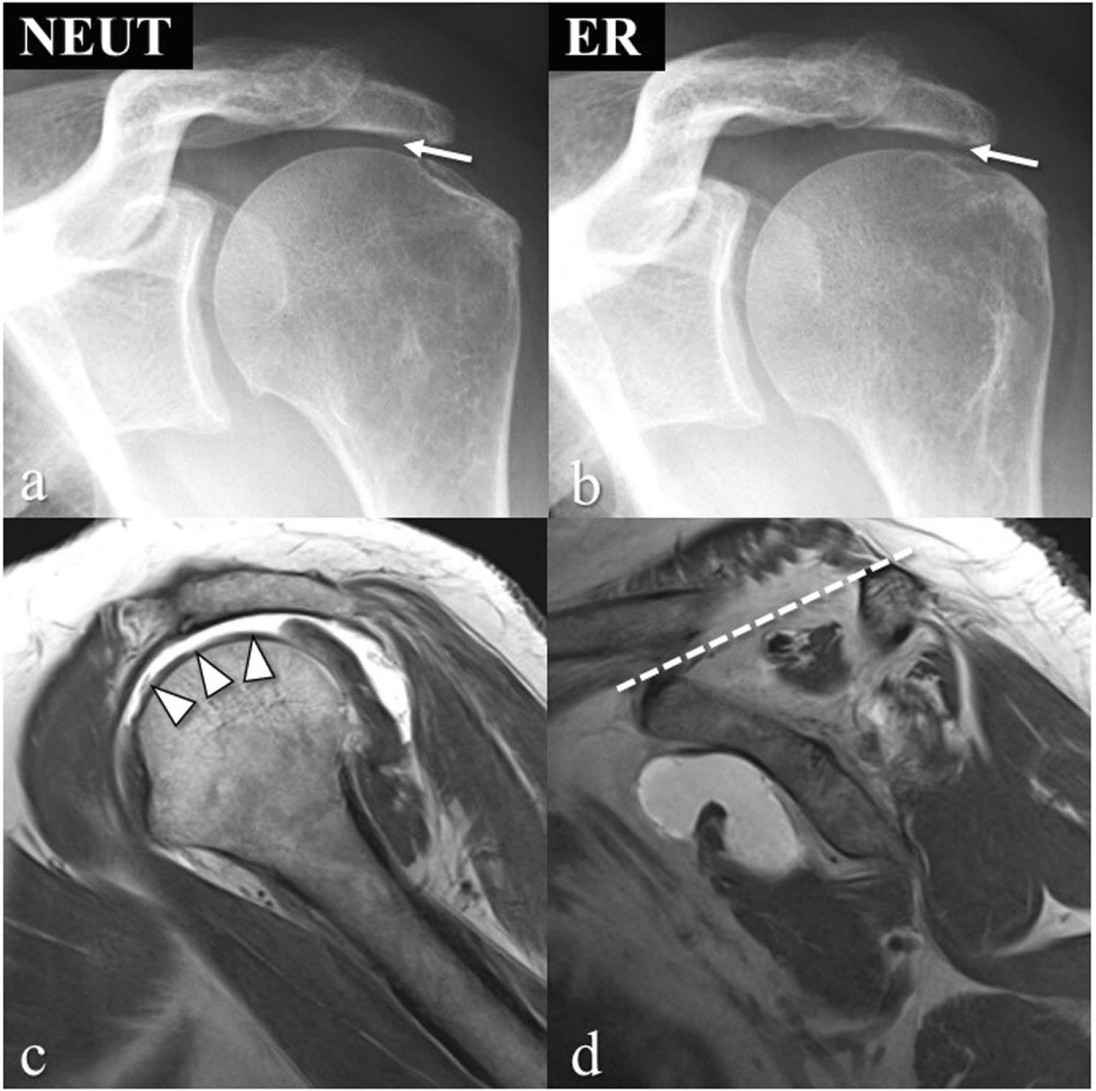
Massive rotator cuff tear in the left shoulder of a 56-year old woman. a, b Oblique anteroposterior radiograph in neutral position (NEUT) shows small acromiohumeral distance (ACHD) as indicated by the arrow, with no change in external rotation (ER). c Sagittal T1-weighted MR image depicts a complete tear of the supraspinatus tendon (arrowheads). d Sagittal T1-weighted MR image shows a positive tangent sign of an atrophied supraspinatus muscle; i.e., the muscle does not cross the dotted line connecting the superior border of the coracoid process to the superior border of the scapular spine
